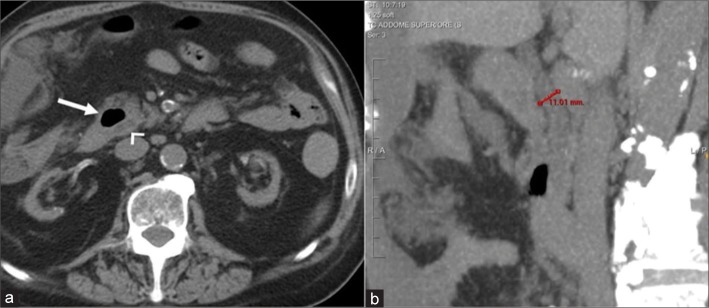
A 79-year-old man presented with epigastric pain. Unenhanced computed tomography scan of the abdomen demonstrated a 27-mm periampullary duodenal diverticulum filled with air (arrow in a) strictly adjacent to the distal portion of the common bile duct (arrowhead in a) which is 11 mm in maximum diameter (b). Symptoms regressed after NG decompression.
